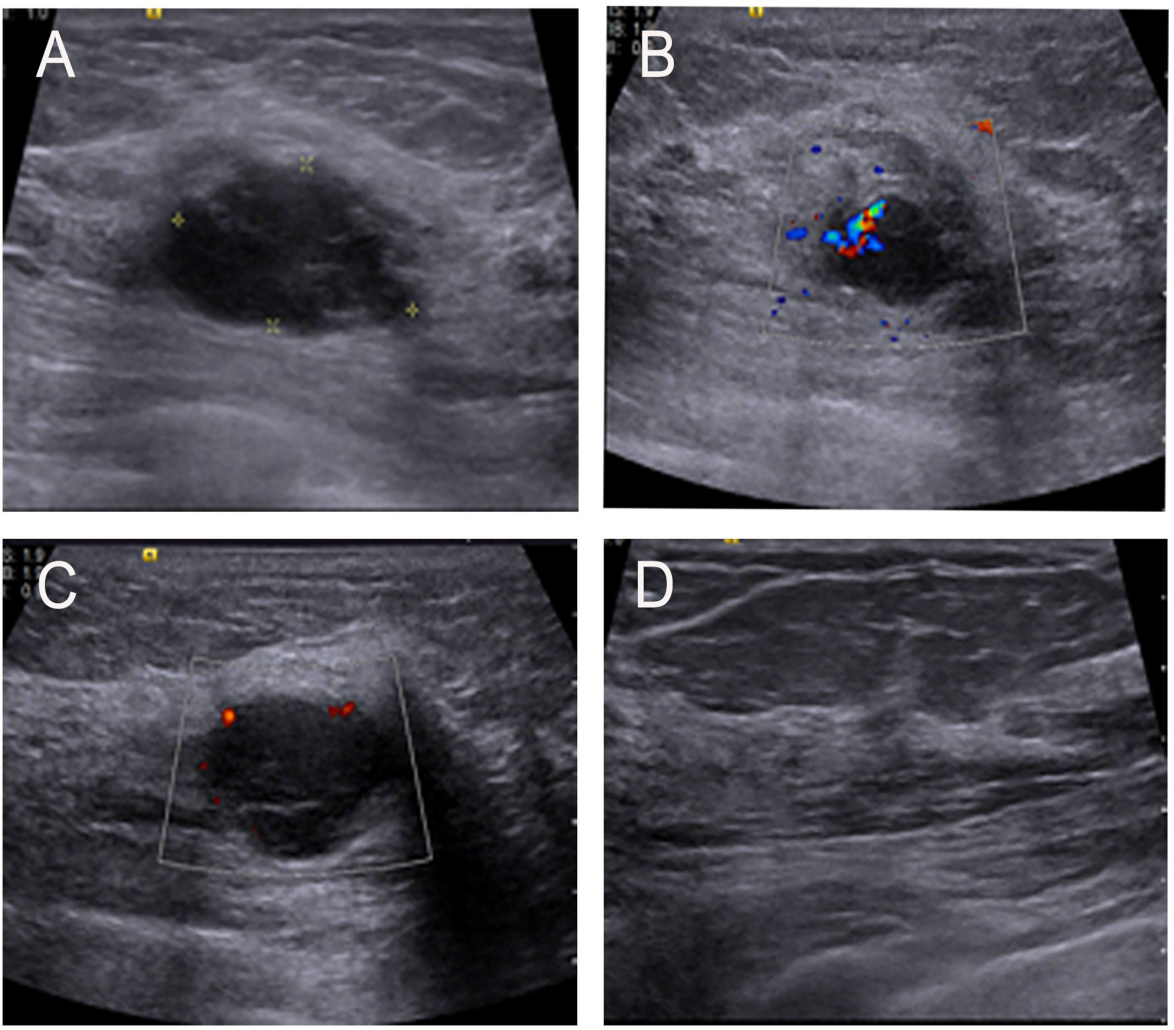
(A) Ultrasonographic image showing a low echo mass in the upper inner quadrant of the left breast and with an irregular shape and uneven internal echogenicity. (B, C) Color-flow Doppler image showing small amount of blood flow signals inside and vessels in rim. (D) No enlarged lymph nodes were found in the armpit.
